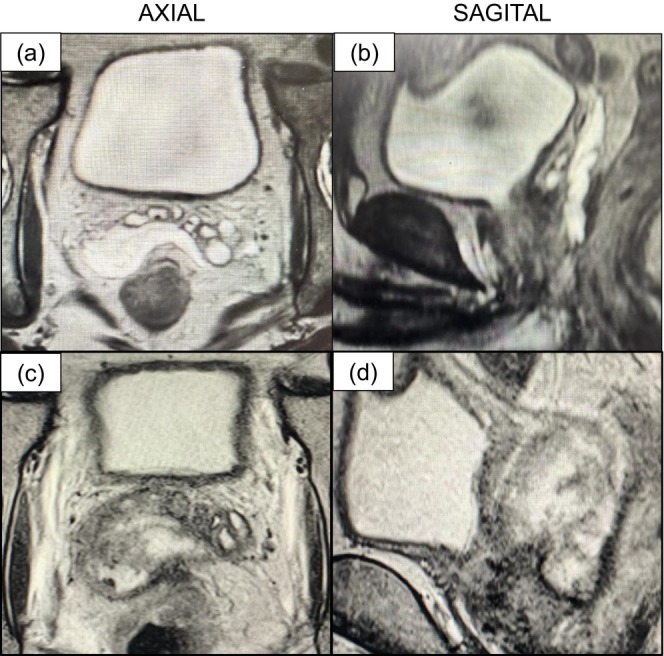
MRI images—comparison before and after infection. (a, b) MRI image after SpaceOAR placement. (c, d) MRI images at the time of infection.
